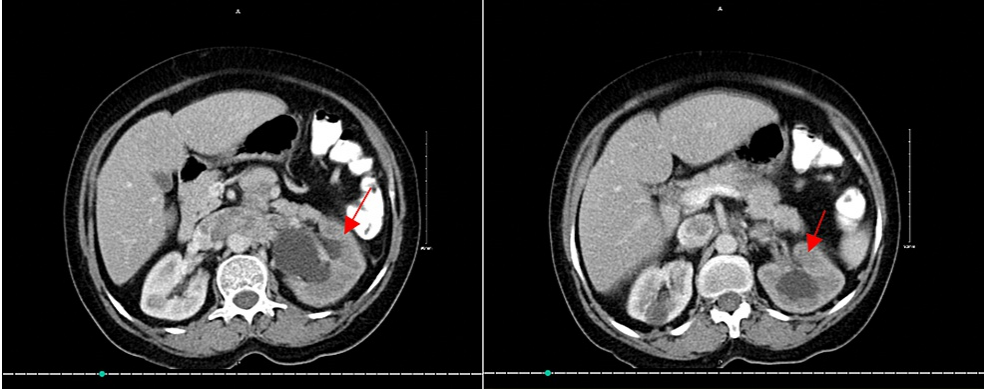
CT scan (axial view) showing Leiomyosarcoma as pointed by red arrows. Left-hand side image: Superior cut; Right-hand side image: Inferior cut
